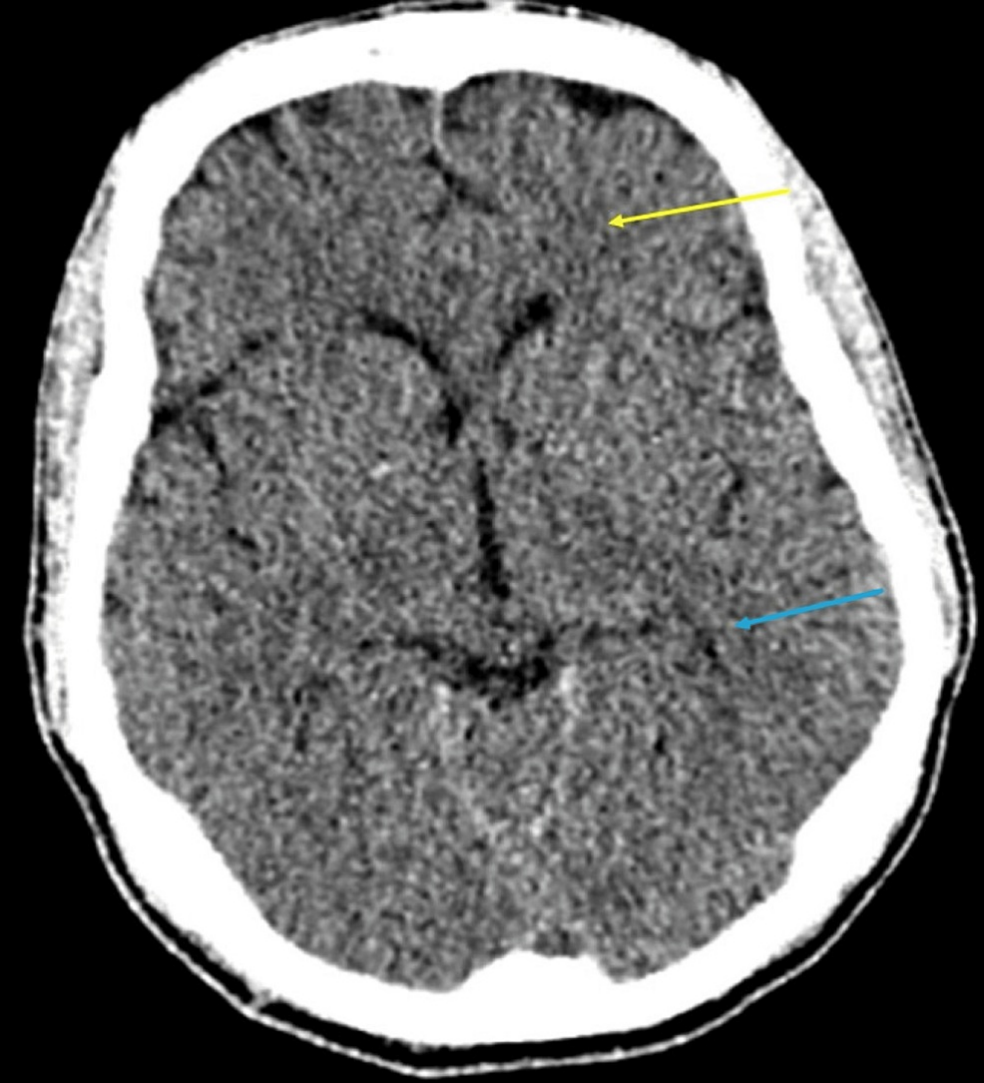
CT head with ill-defined hypodensities in the left frontal (yellow arrow) and parietal lobes (blue arrow) concerning acute ischaemic infarcts
